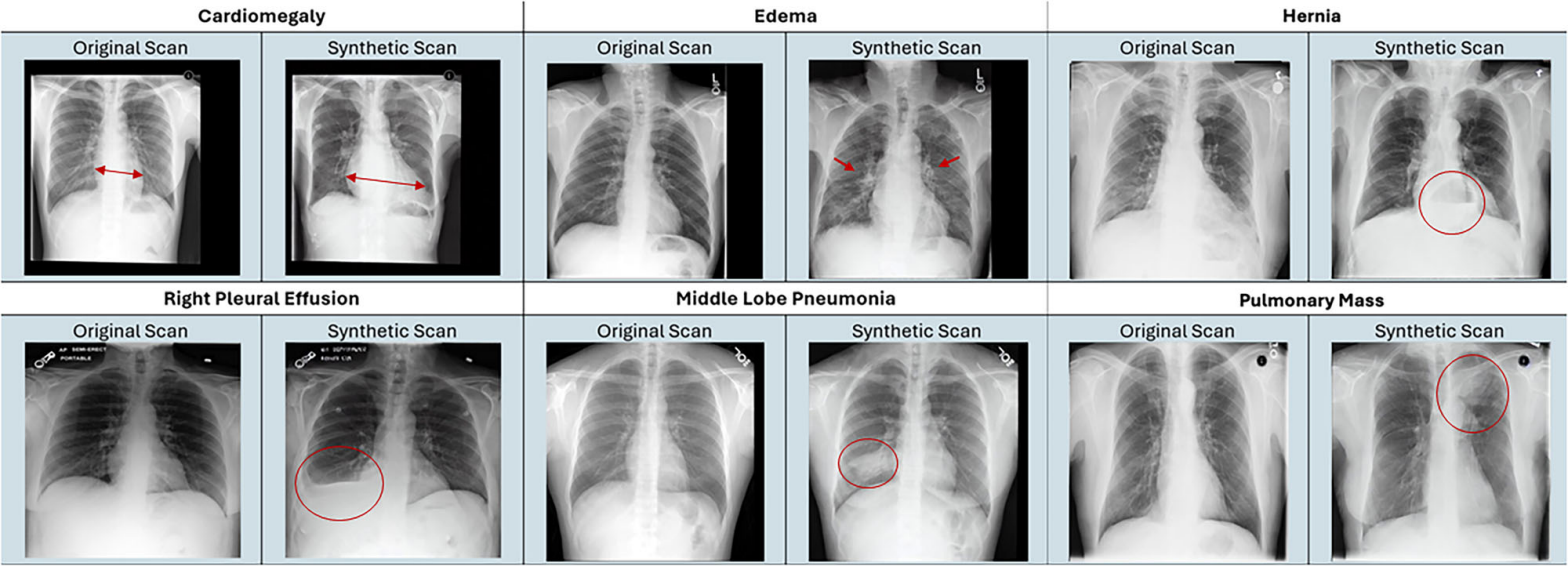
Representative real and counterfactual annotated chest radiographs produced by RoentMod. Each panel represents a disease RoentMod was prompted to introduce. The left image within the panel represents the original scan with no marked findings, while the right image within the panel represents the RoentMod-modified synthetic scan that contains the introduced finding. The red markings represent the locations of each introduced finding, while the red mark on the original scan for cardiomegaly represents a reference smaller heart size that makes the cardiomegaly more apparent in the synthetic scan.
